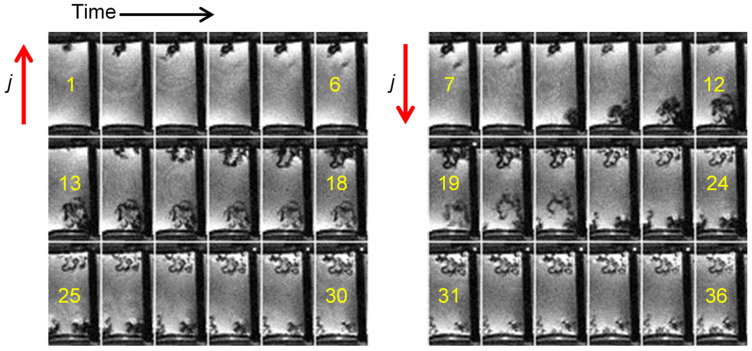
Time-lapse MR images of a representative cross section through a symmetrical lithium cell. The cell was charged and then discharged (by reversing the current) every six frames (20 h). The charging regime (*j* = 0.5 mA/cm^2^, Sand’s time of 38.2 h) was such that the experiment was performed in the reaction-limited regime. The experimental conditions were favourable for the growth of lithium whiskers, which is clearly visible from frame 10 onwards. In frames 1–6, the anode is the top electrode and dendrites are formed there first. When the current is reversed (in frames 7–12), the dendrites in the top electrode begin to decompose and almost disappear by frame 12, while they start to grow on the new anode (bottom electrode). A similar effect, but to a lesser extent, is also visible in later frames with each change in the direction of the current.
